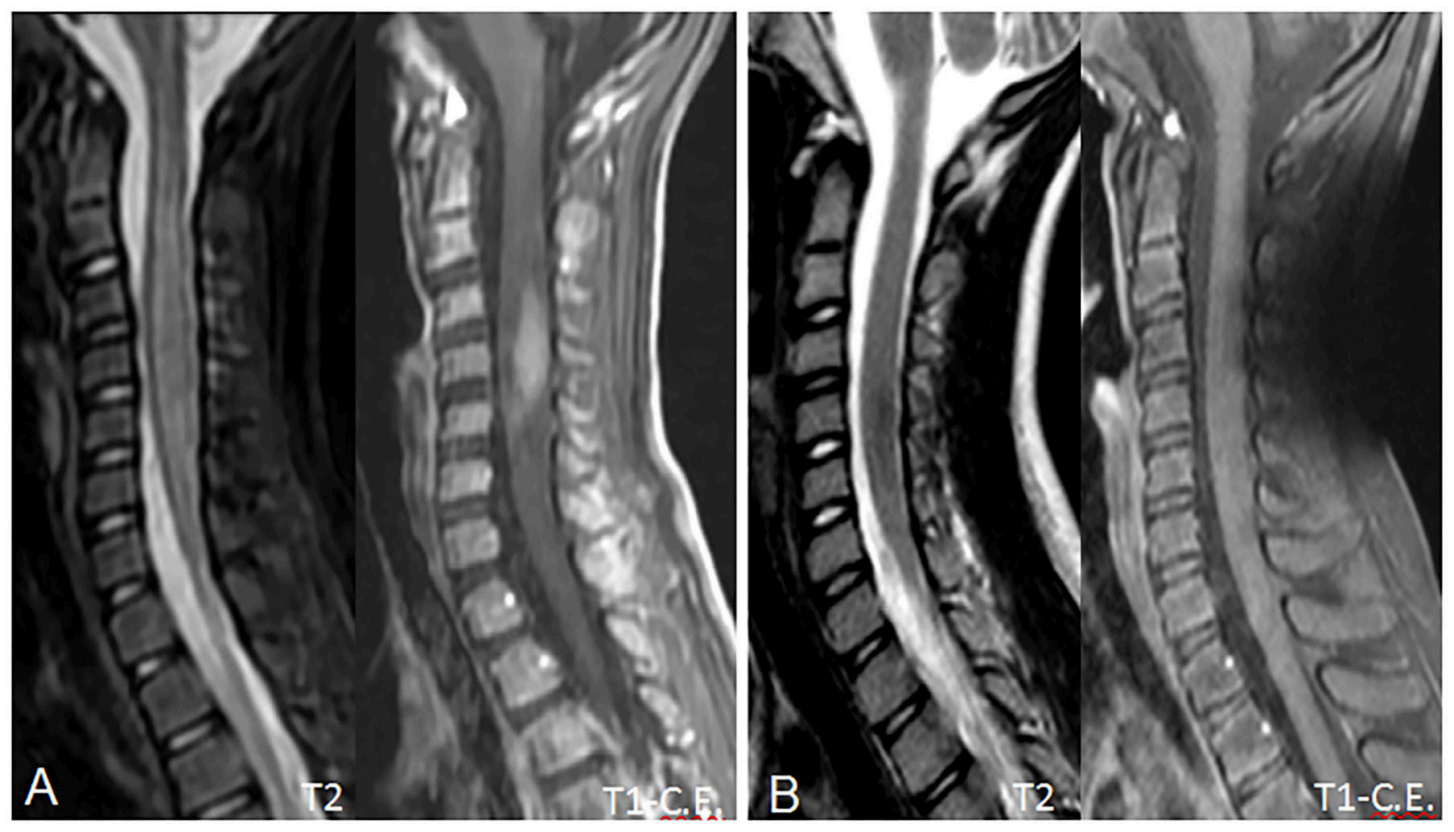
Follow-up MRI scans at 20 (A) and 55 (B) days, showing progressive reduction of the spinal cord swelling and contrast enhancement. In (B), contrast enhancement has completely regressed, due to blood-brain barrier restoration, and the spinal cord shows a near-normal appearance with no residual disease. No spinal cord atrophy is noted.
